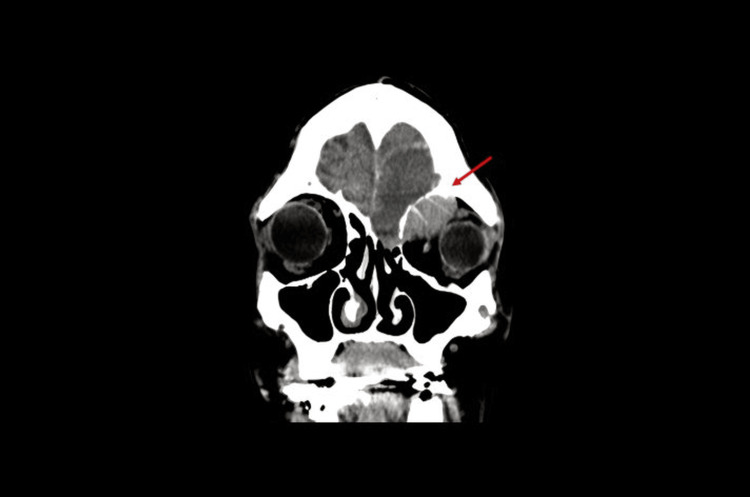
Cranial and paranasal sinuses CT scan, coronal section Coronal CT scan showing a heterogeneous extraconal soft tissue lesion in the left orbital cavity (31 × 31 × 36 mm), located in the superolateral quadrant, with poorly defined margins and significant inferomedial displacement of the ipsilateral globe, consistent with proptosis.
